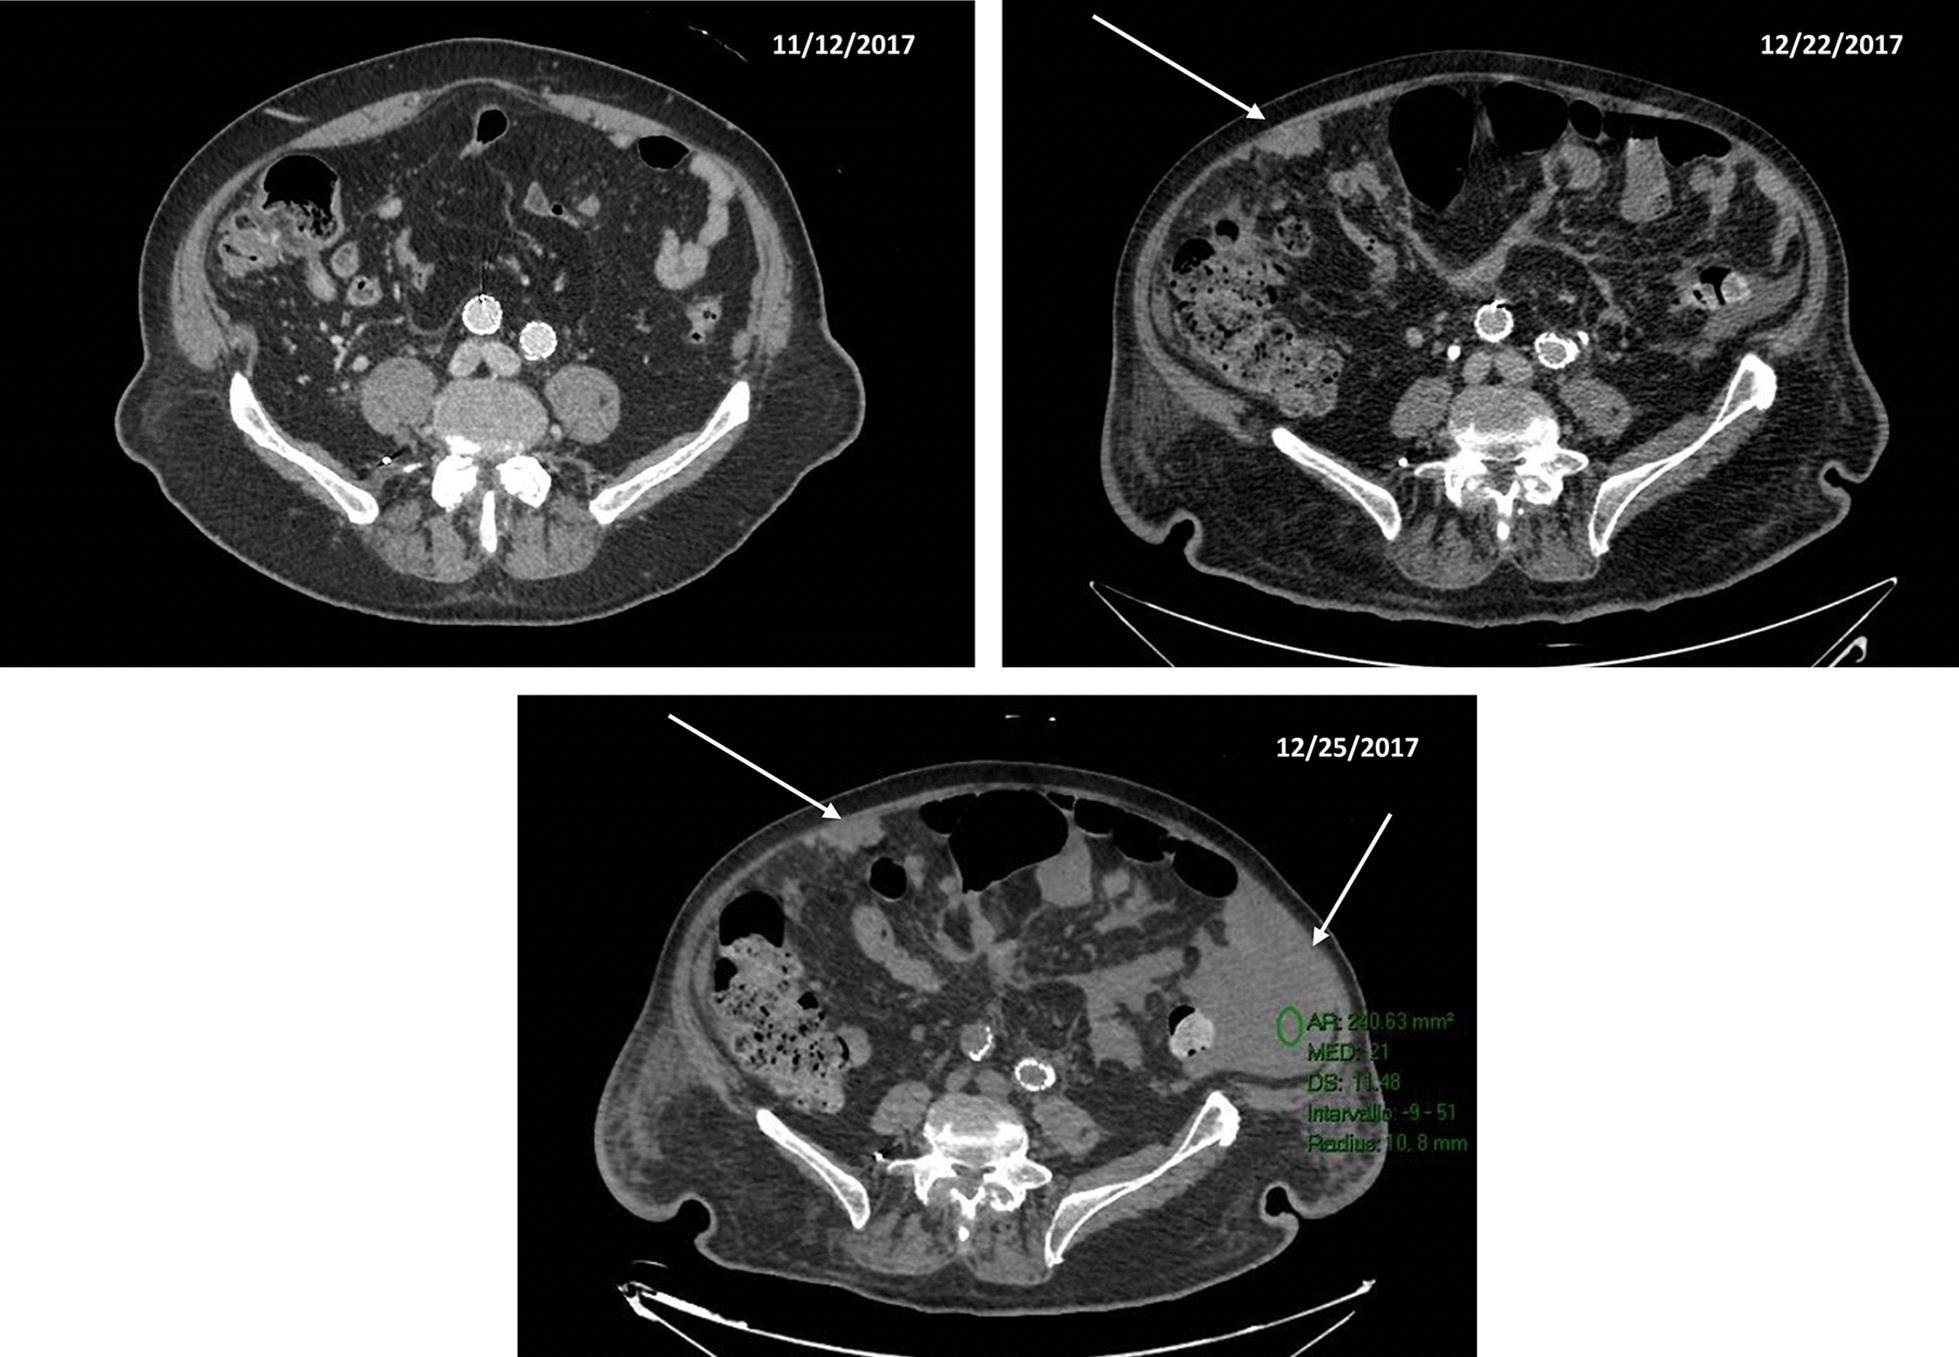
CT imaging showing the rapid evolution of the peritoneal localizations and ascites
